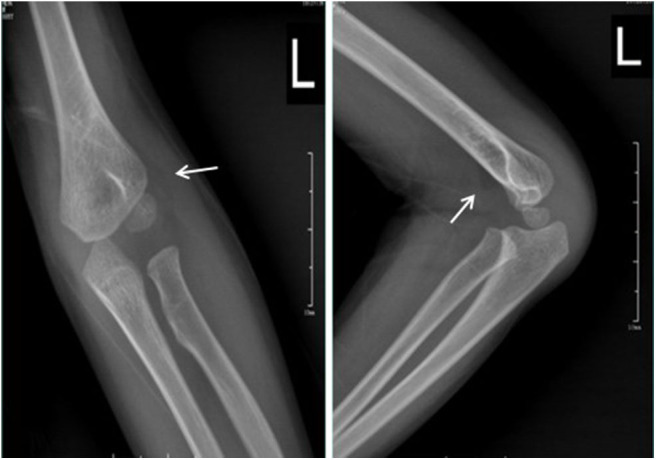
Standard radiographs in AP and LL projections showing anterior and posterior fat pads (arrow).
